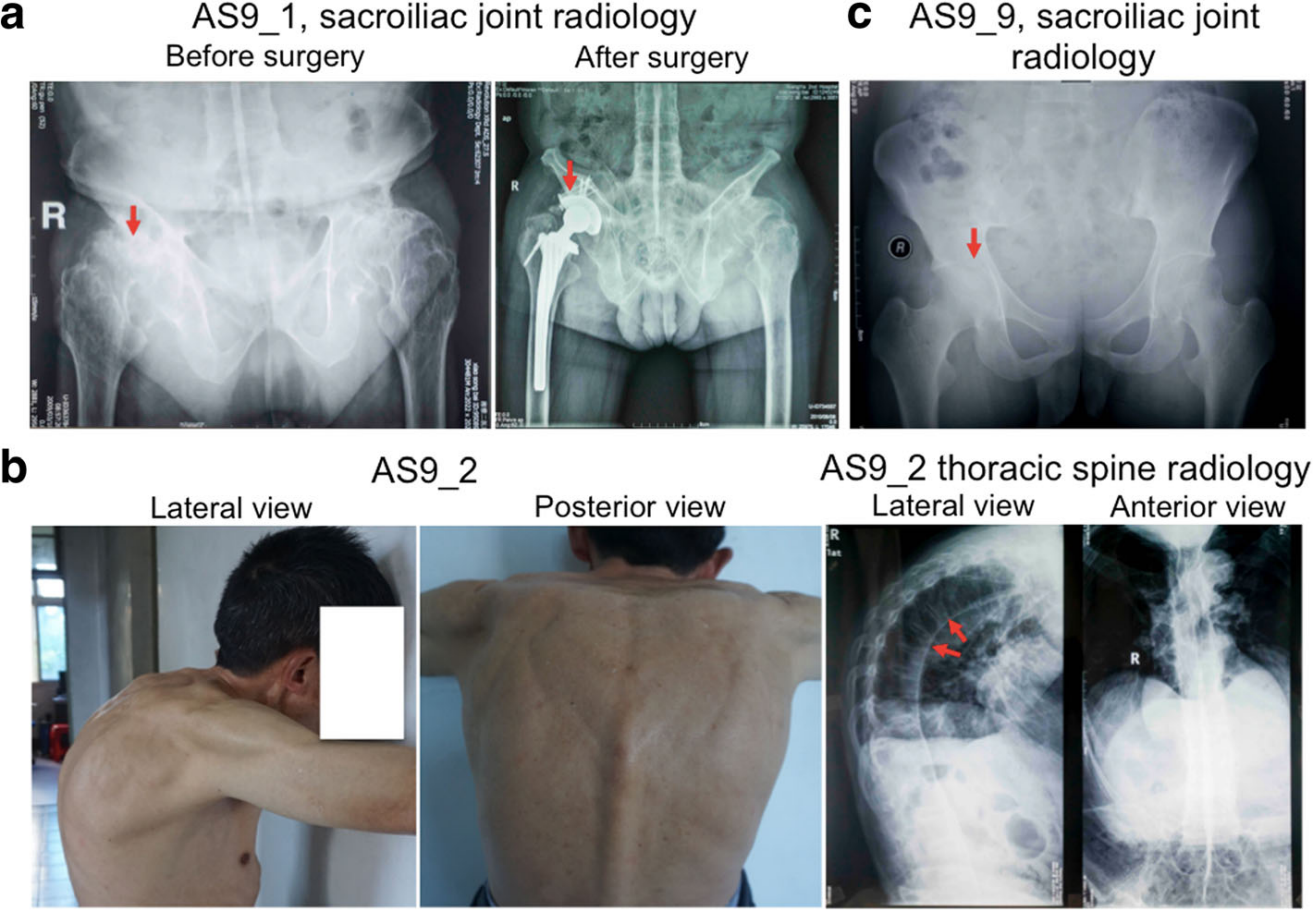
Medical images of AS9 patients. a X-ray pictures of the sacroiliac joints of the proband, AS9_1, before (left) and after (right) joint replacement surgery. Arrows indicate erosion of the right joint before the surgery (left) and the artificial joint after the surgery (right). b Medical images of patient AS9_2, showing the deformation of the thoracic spine due to ankylosis (left). X-ray pictures showing the “bamboo”-like spines of AS9_2 (right). Arrows point to the sites of fused vertebrae. c Sacroiliitis of patient AS9_9 detected by X-ray photography
